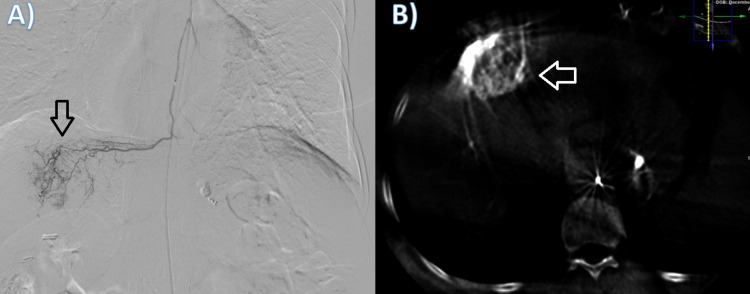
A: DSA of the left internal mammary artery showing tumoral blush supplied by distal branches. B: axial CBCT scan was obtained during the procedure confirming the tumoral supply by left internal mammary artery. DSA, digital subtraction angiography; CBCT, cone beam CT
